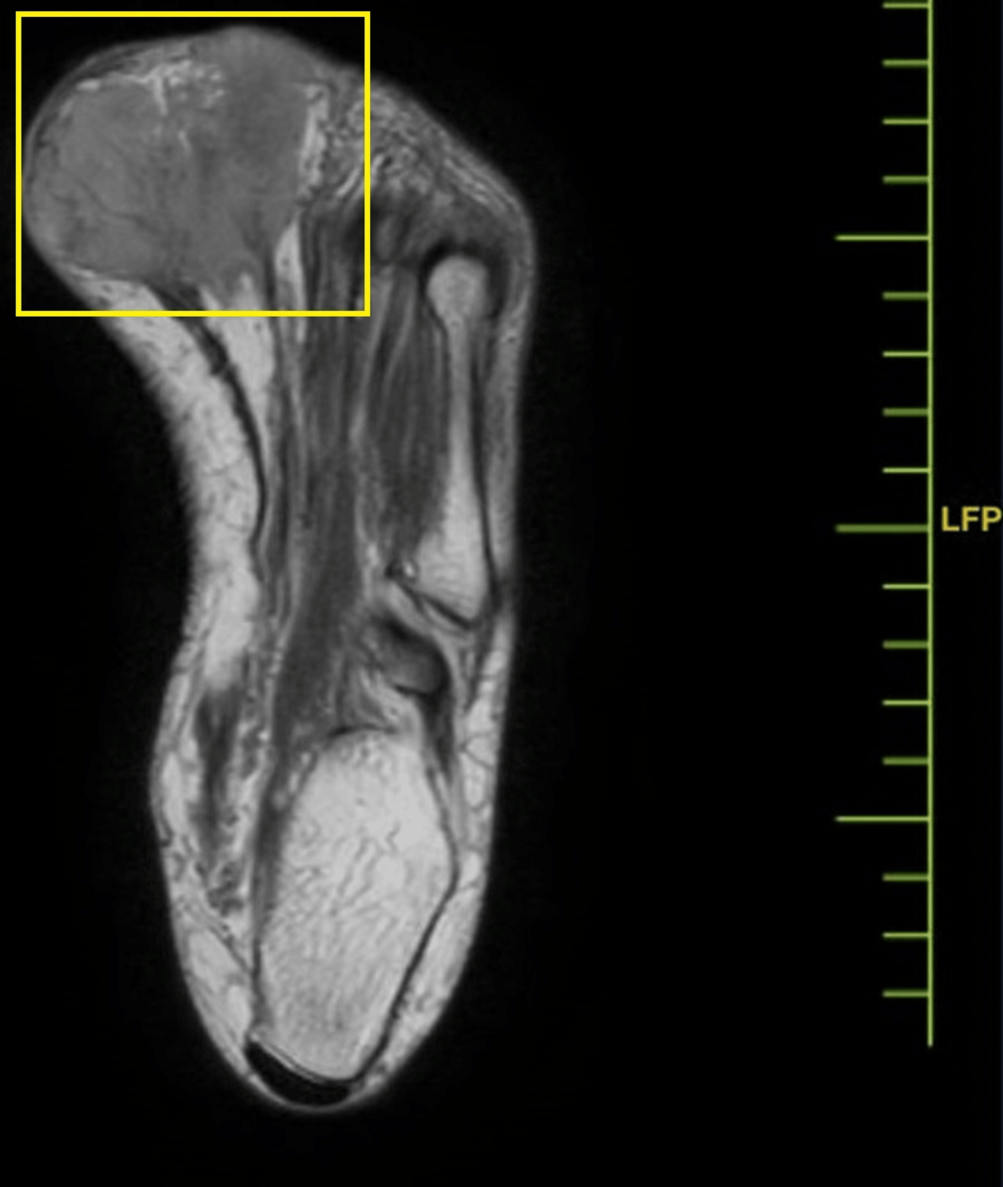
MRI of the foot (coronal plane) MRI: magnetic resonance imaging
